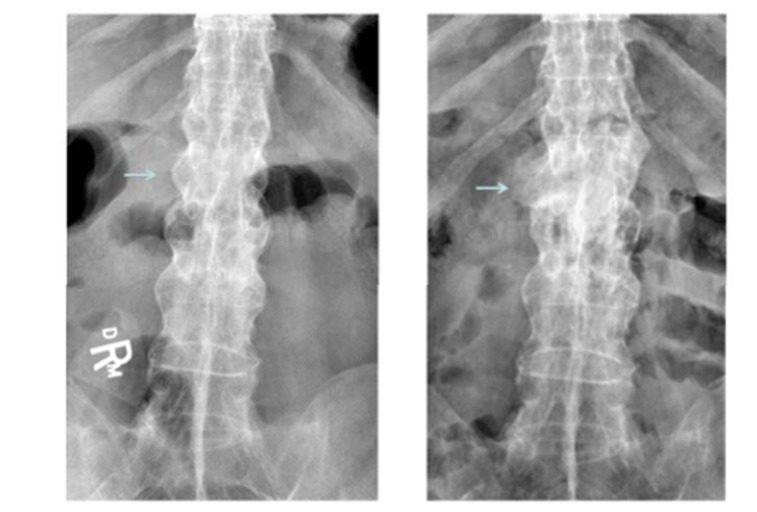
Arrows pointing to the fracture areas on the initial AP xray and follow up AP xray.
